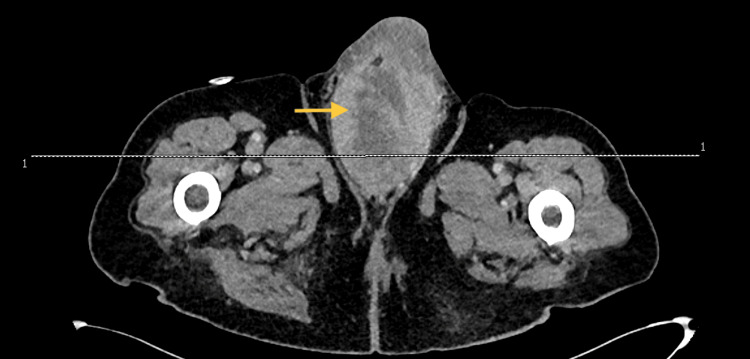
CT scan representing abnormality involving root of penis at subsequent presentation
